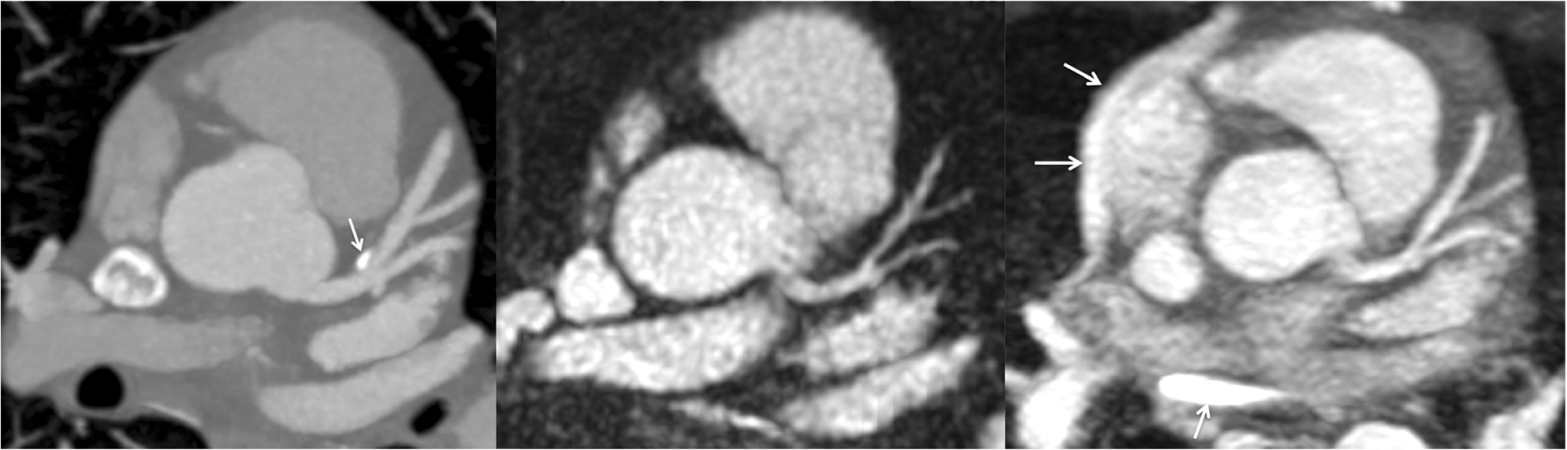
39-year-old male evaluated for chest pain. *Left*: 5-mm MIP of coronary CT angiography shows mild narrowing of the proximal LAD and a punctate coronary calcification (arrow). Middle: MIP of breath-hold radial QISS MRA obtained at 1.5 Tesla demonstrates the left main and LAD coronary arteries, including the D1 and D2 branches. The appearances are similar to the coronary CT angiogram except that the wall calcification is not visible. *Right*: MIP from navigator-gated 3D bSSFP also demonstrates the left coronary anatomy comparably to the coronary CTA. Compared with QISS, there is increased pericardial fluid signal (arrows)
